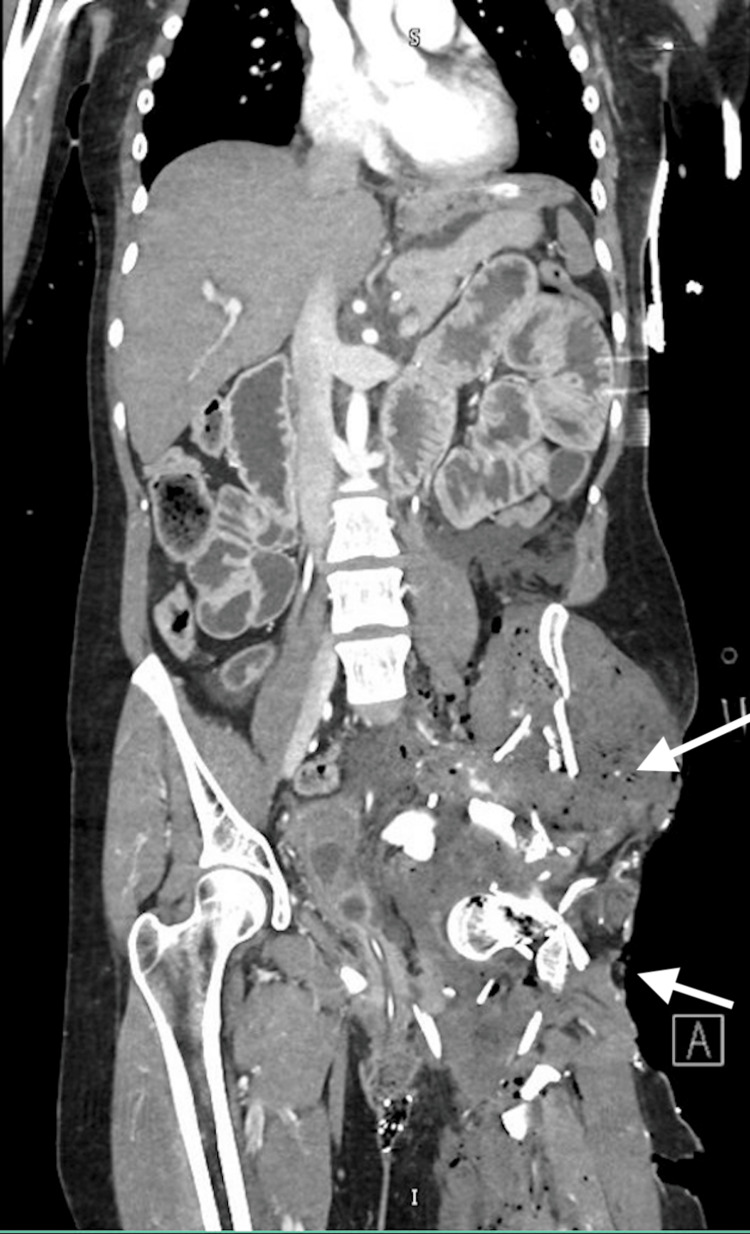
CT scan of the abdomen and pelvis with IV contrast The arrows indicate destruction of the hemipelvis and severe soft tissue injury.
